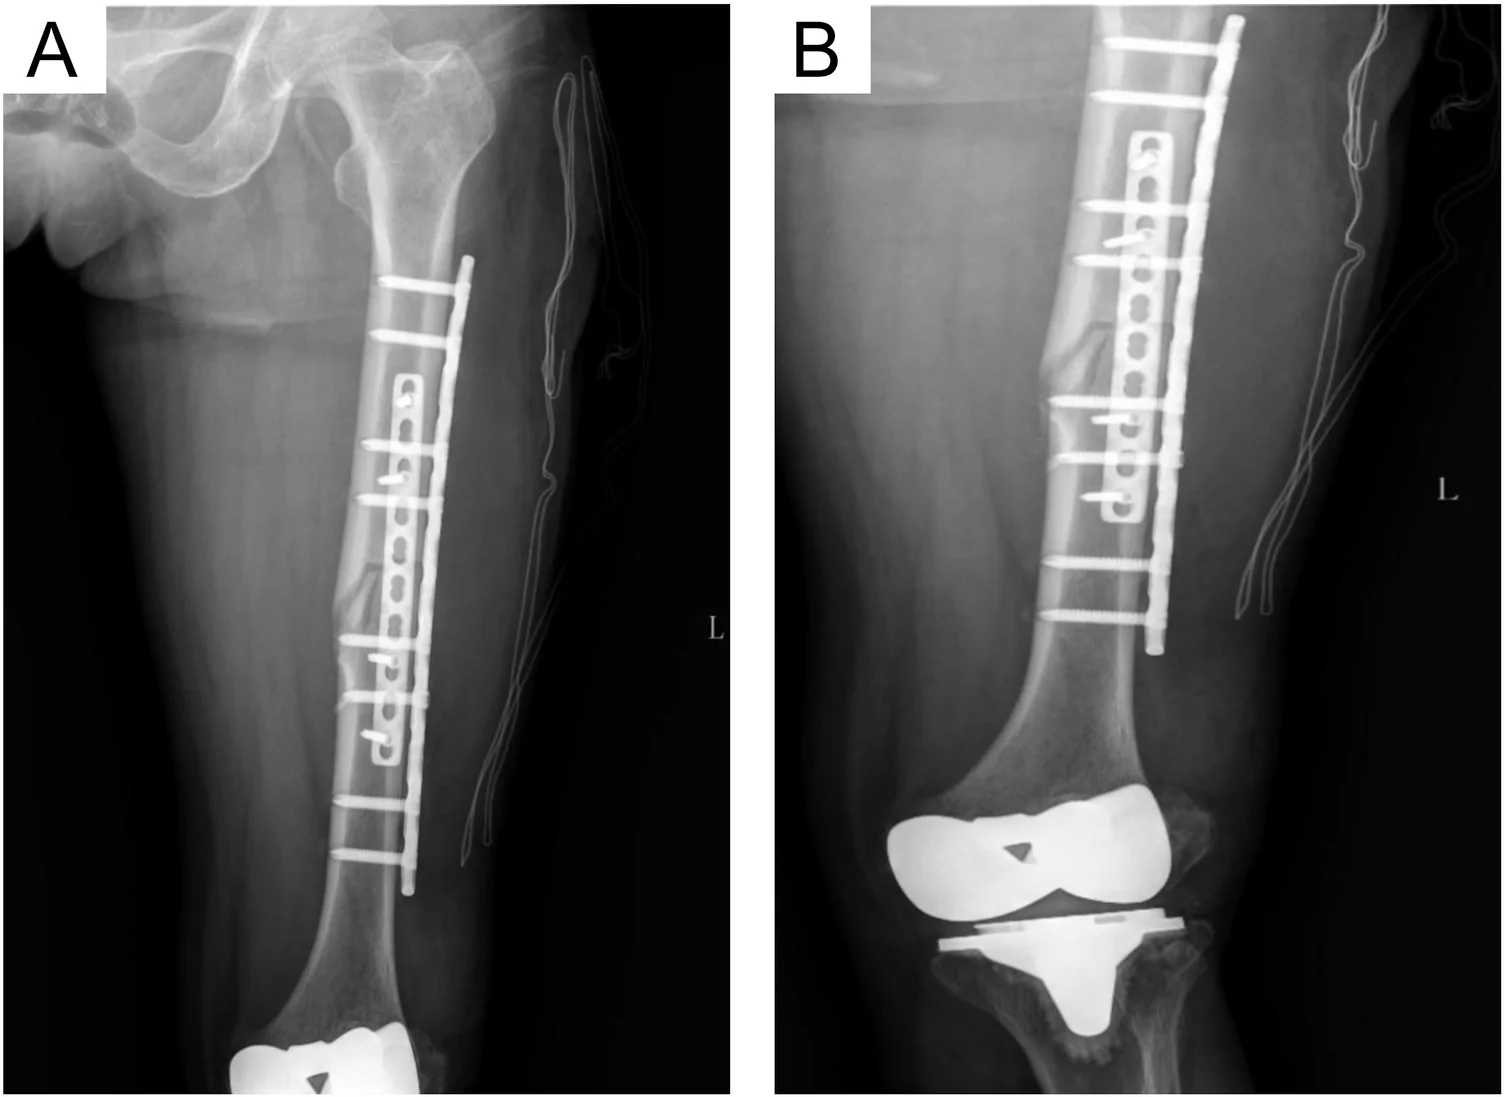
One-week postoperative full-length anteroposterior radiograph of the left femur. (A,B) The images demonstrate stable internal fixation and anatomical alignment achieved via extramedullary bone plating, successfully bypassing the fracture line and the occult bone cement plug site.
